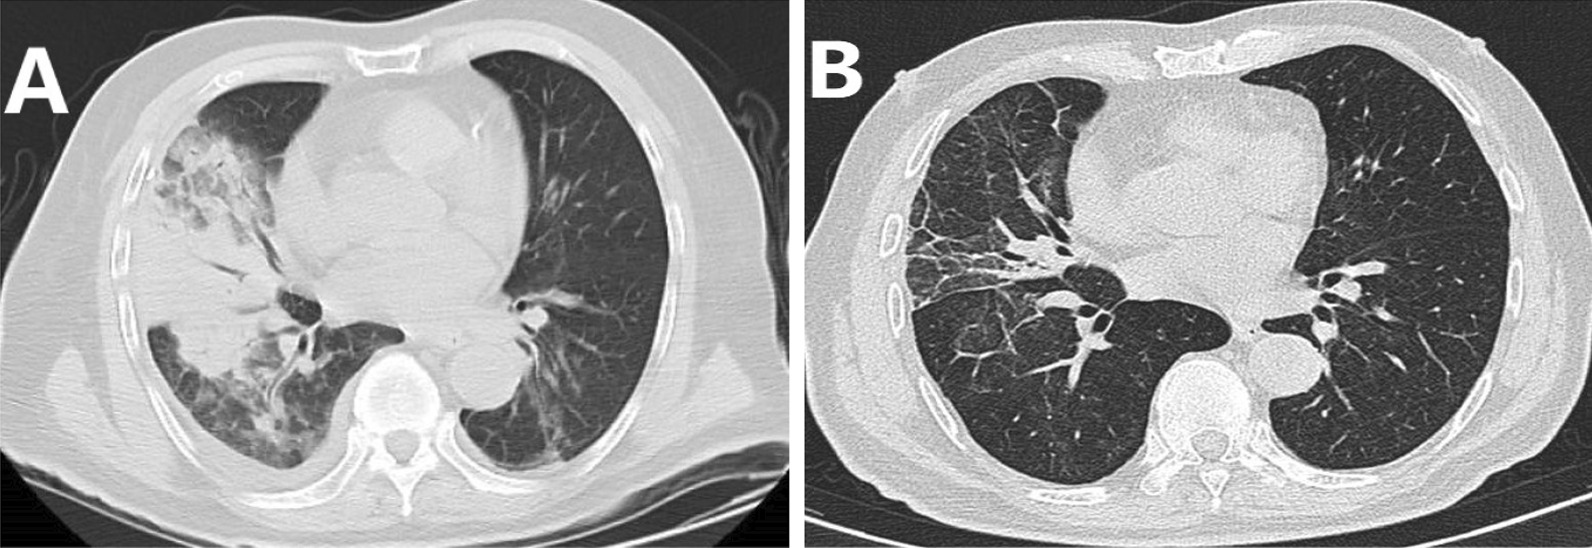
Chest computed tomography (CT) scans of a 78-year-old man with severe *psittacosis* pneumonia. The initial CT scan (11 days after onset) shows air-space consolidation with inflammatory exudation in the superior lobe of the right lung and pleural effusion (A). On follow-up, the consolidation was completely absorbed (19 days after onset) (B)
